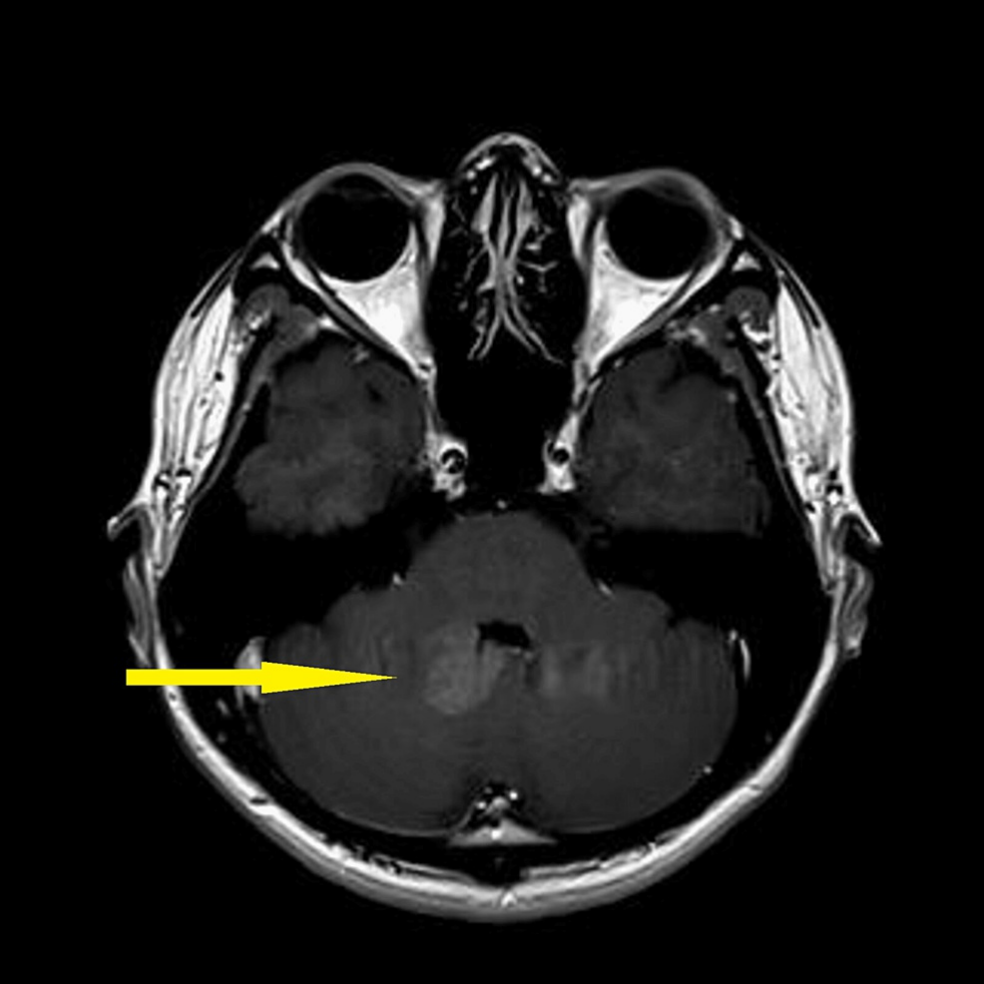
Enhanced MRI brain (T1) axial post-contrast showing an ill-defined lesion in the fourth ventricle (yellow arrow)
